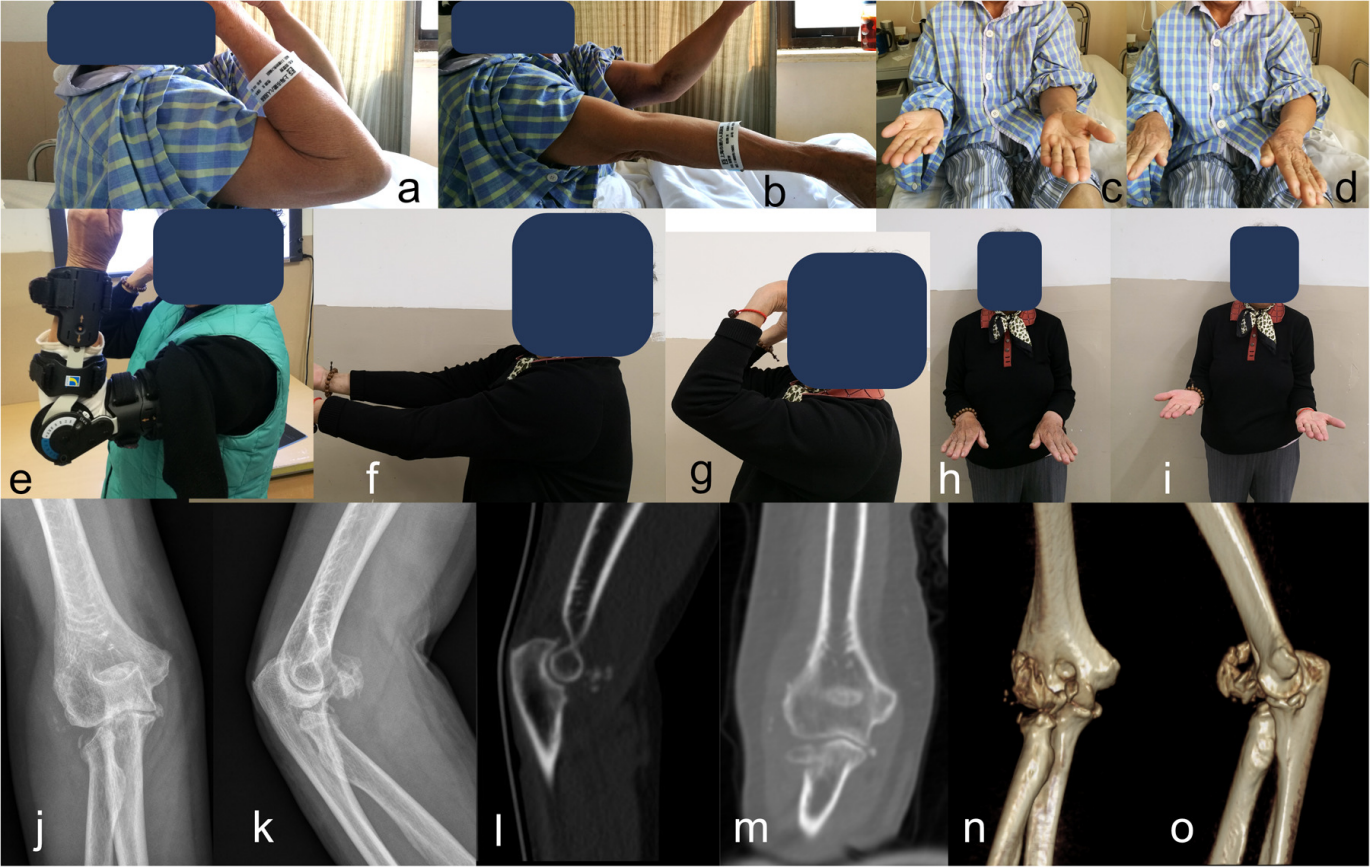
Non-operative treatment of the patient whose fracture patterns are shown in Figure 1. Elbow extension and pronation were limited after the injury (a–d). Though dislocated, the elbow joint instability was relatively easy to regain after reduction in the valgus-type TTI; elbow brace was used to facilitate rehabilitation (e). Joint function was improved after 12 months (f–i); radiographs show the radial head malunion, non-union of MCL avulsion, and lateral heterotopic ossification (j–o).
